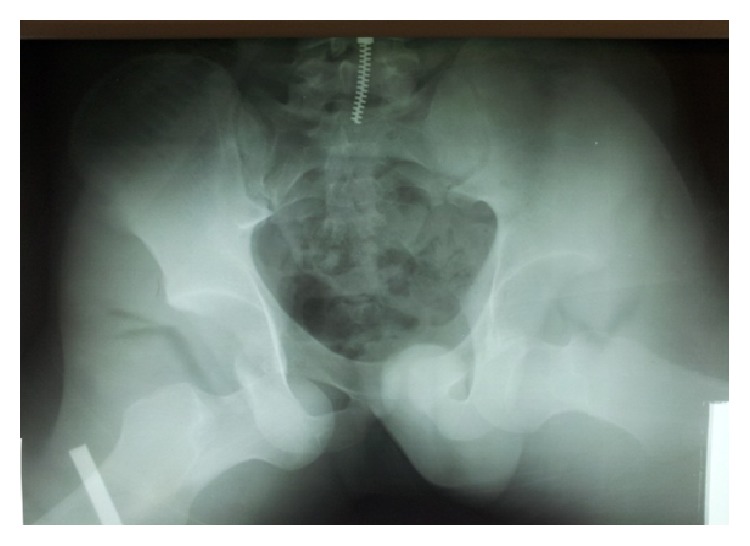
X-ray of a bilateral simultaneous traumatic obturator dislocation of both hip joints.
